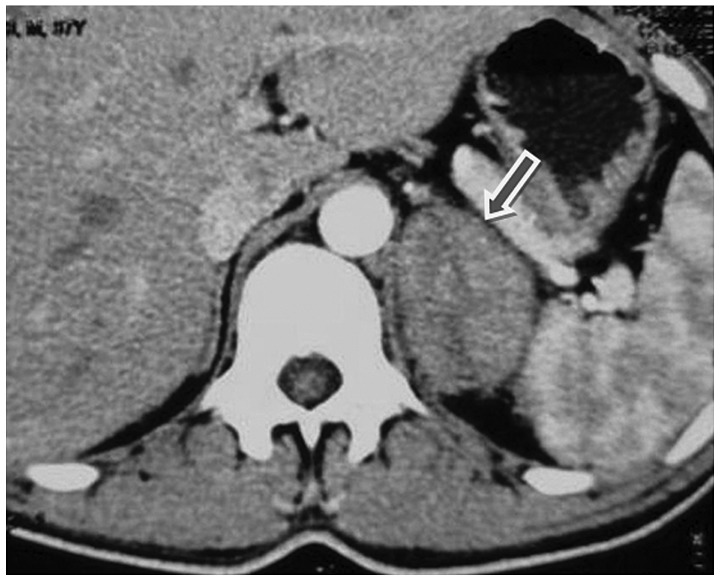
Abdominal computed tomography of the patient with primary adrenal nodular lymphocyte-predominant Hodgkin lymphoma. A soft tissue mass was observed in the left adrenal gland (arrow), measuring 4.5×5.5 cm, with a less uniform density and clear borders.
